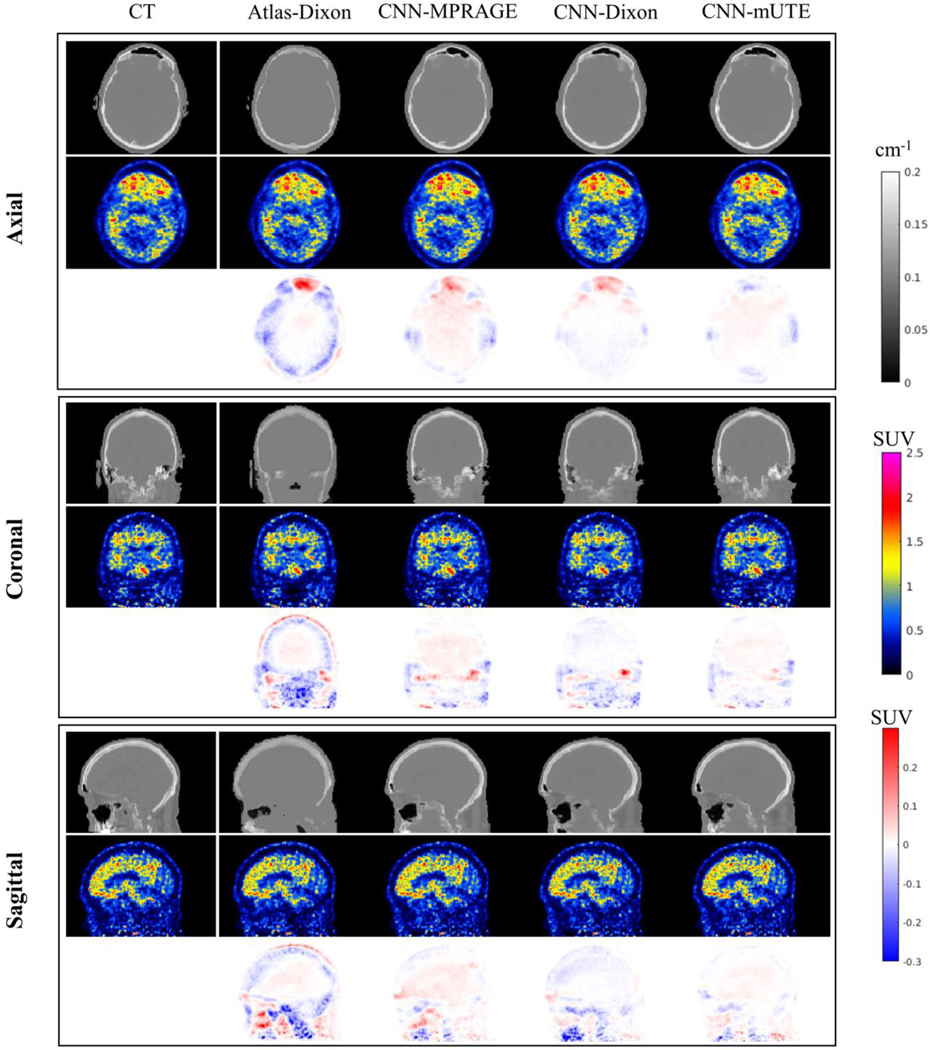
Three views of the CT/pseudo-CT images, PET images (^11^C-PiB, unit: SUV) and the corresponding PET error images (*PET_pseudoCT_* − *PET_trueCT_*, unit: SUV) for one subject. For each view, the first row shows the ground-truth CT image (first column) and the pseudo-CT images generated by Atlas-Dixon (second column), CNN-MPRAGE (third column), CNN-Dixon (fourth column) and CNN-mUTE (fifth column), respectively; the second row shows the PET images reconstructed using the ground-truth CT image and different pseudo-CT images; the third row shows the corresponding PET error images for different methods.
